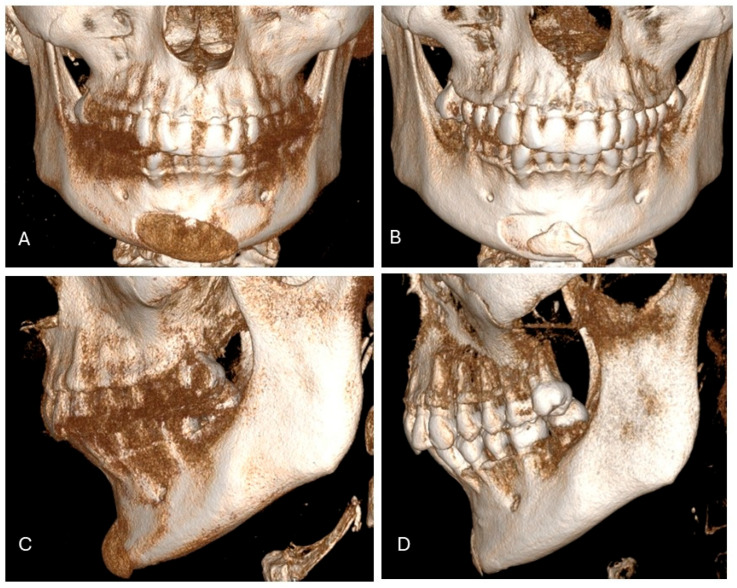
A 42-year-old Caucasian woman presented for consultation because of a mispositioned chin implant placed elsewhere four years earlier (A). She complained of asymmetry, palpable implant mobility, tissue irritation, and mild pain. CT showed implant malposition due to lack of fixation (A,C), with bone resorption and ectopic bone formation (B,D). The patient was scheduled for implant removal with immediate osteoplasty and placement of a custom-made PEEK chin implant.
